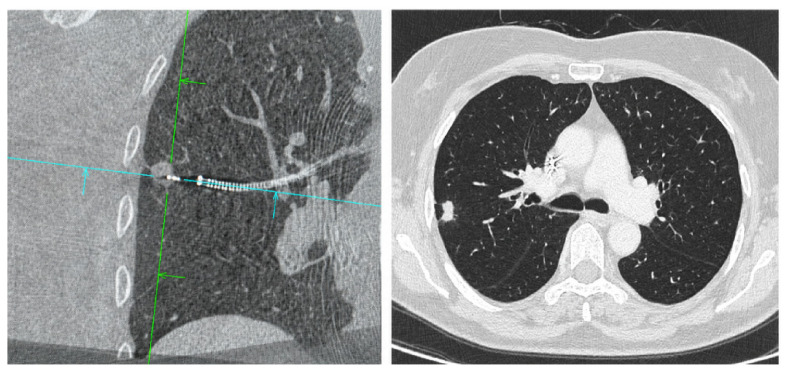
Representative intraprocedural and radiological imaging of a right upper lobe pulmonary nodule. (Left) Tool-in-lesion confirmation with the cryoprobe accurately positioned within the target lesion during shape-sensing robotic-assisted bronchoscopy (ssRAB) performed with the ION^TM^ Endoluminal System. (Right) Axial chest CT image in the lung window setting demonstrating the corresponding pulmonary nodule in the right upper lobe.
